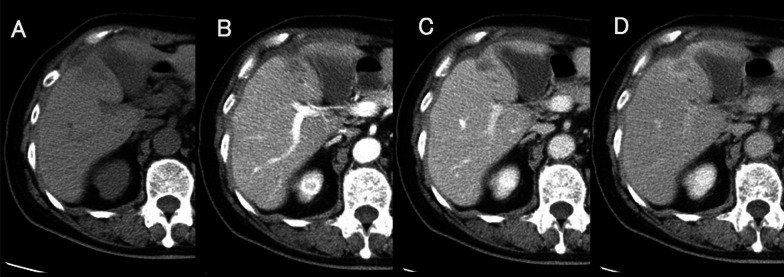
Abdominal computed tomography images after drainage and chemotherapy (A plain, B arterial phase, C portal phase, and D equilibrium phase)
